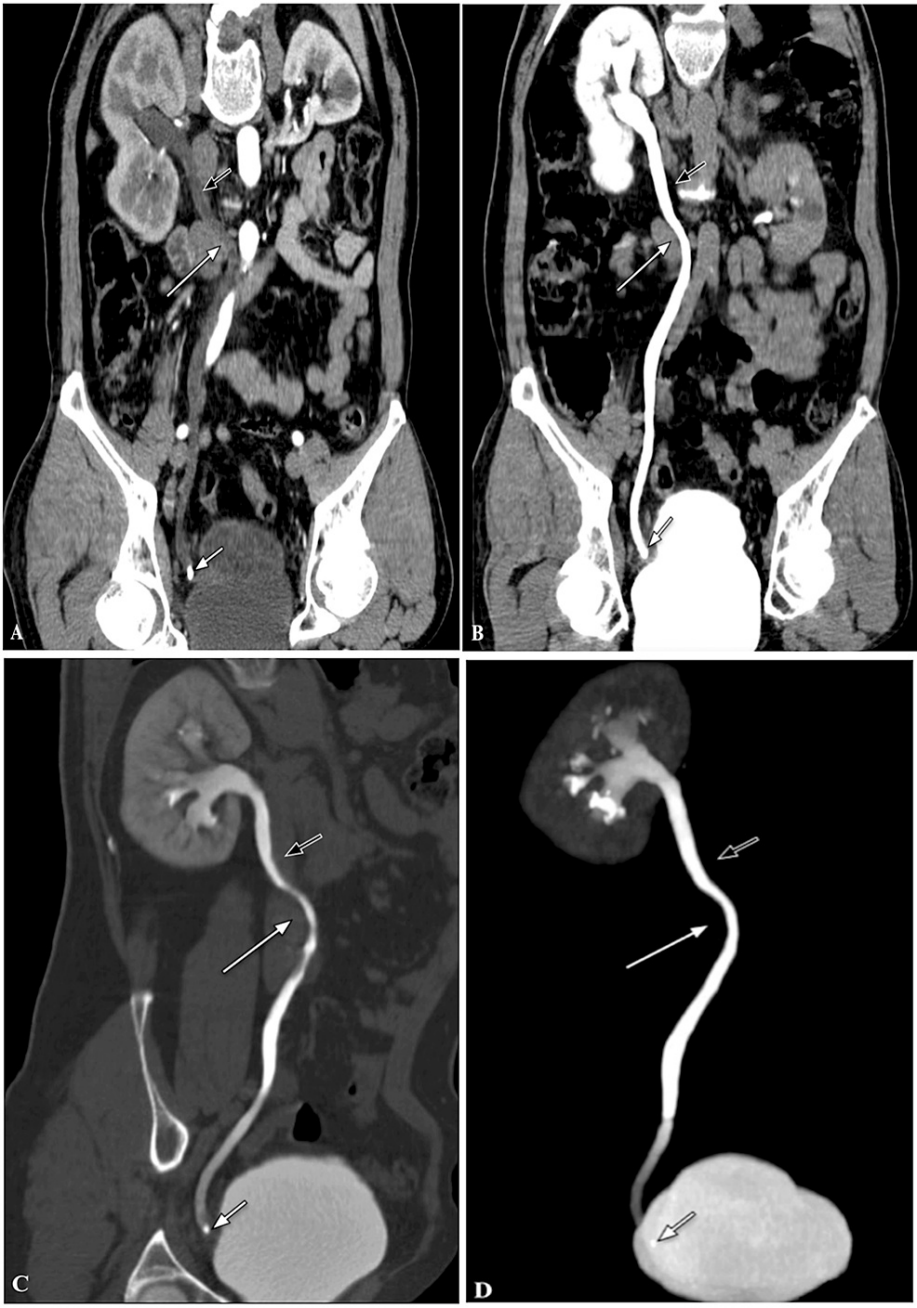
CTU with 3D curved coronal MPR (A) Venous phase, (B, C) Delayed phase, and (D) Delayed phase with Coronal Maximum Intensity Projection shows the medial deviation of the right mid-ureter with an “S”-shaped deformity of the right proximal ureter, and a radio-opaque calculus in the right distal ureter (close to the vesicoureteric junction), causing mild mid and distal hydroureter. Note: Right proximal ureter (short black arrow), right mid-ureter (long white arrow), calculus (short white arrow). CTU: Computed tomography urography, 3D: Three-dimensional, MPR: Multi-planar reconstruction.
